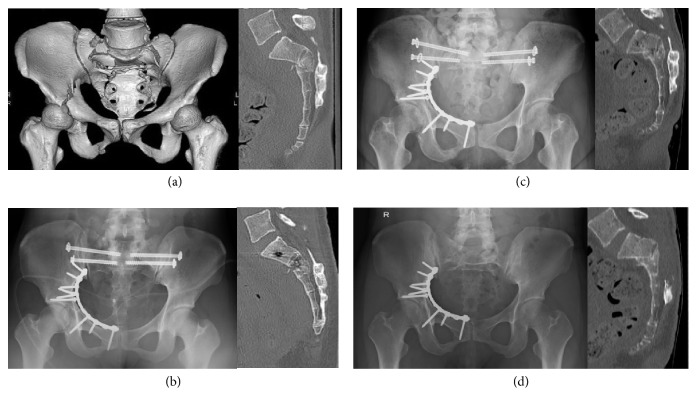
Case 3. This case had the greatest loss of reduction. A 25-year-old woman who was injured from a fall from a height underwent unilateral S1 and S2 bilateral iliosacral partial threaded screw fixation for a Roy-Camille type 2 H-shaped transverse sacral fracture. (a) Preoperative 3-dimensional and sagittal plane CT reconstruction of the pelvis is shown (preoperative sacral kyphotic angle: 63°; fracture translation: 11 mm). (b) Postoperative anteroposterior radiograph and CT scan of the sacrum in the sagittal plane is shown (postoperative sacral kyphotic angle: 28°; fracture translation: 6 mm). (c) The anteroposterior radiograph and CT scan of the sacrum in the sagittal plane taken one month after the initial operative treatment is shown (sacral kyphotic angle: 60°; fracture translation: 14 mm). IS screw loosening was observed. Secondary sacral decompression was performed. (d) Final follow-up anteroposterior radiograph and CT scan of the sacrum in the sagittal plane is shown. Bone healing was achieved, but reduction loss and bladder/bowel dysfunction remained.
